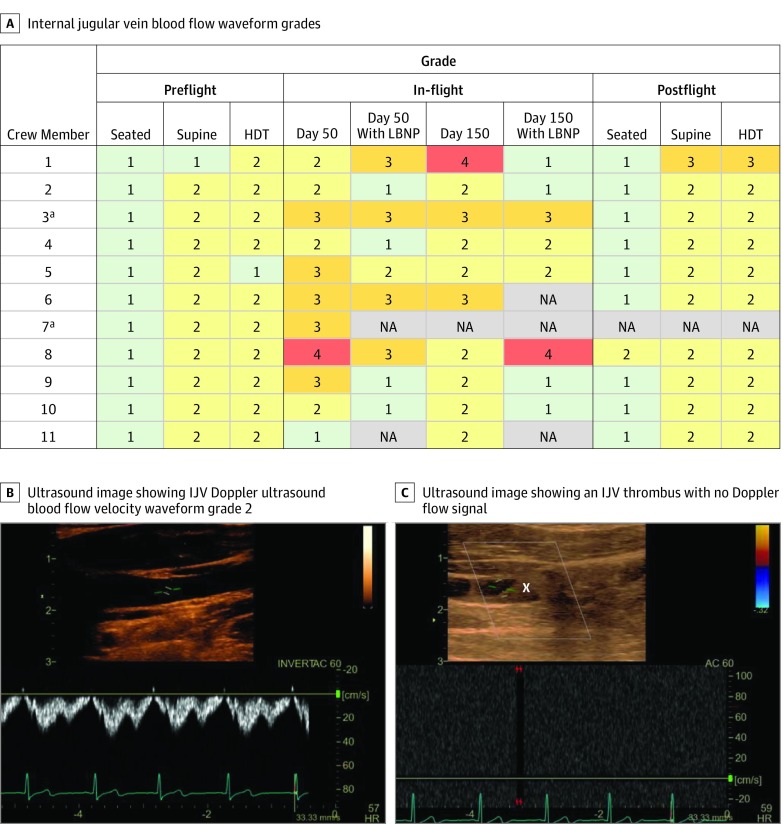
Internal Jugular Vein (IJV) Blood Flow Waveform Grading Before, During, and After Spaceflight and Example Ultrasonography Demonstrating the Presence of a Thrombus During Spaceflight Grade 1 indicates predominantly continuous flow; grade 2, predominantly pulsatile flow that may return to 0; grade 3, no net forward flow (stagnant or equal positive and negative flow); grade 4, retrograde flow. HDT indicates 15° head-down tilt and LBNP, lower body negative pressure (A). Example longitudinal ultrasonographic scans of the left IJV and Doppler ultrasonographic blood flow velocity waveform in one participant showing grade 2 flow preflight measured in the supine position (B), and a thrombus (denoted with an X) with no flow detected through the IJV on flight day 50 on the International Space Station (C). ^a^Denotes crew members who presented with an IJV thrombus during spaceflight.
